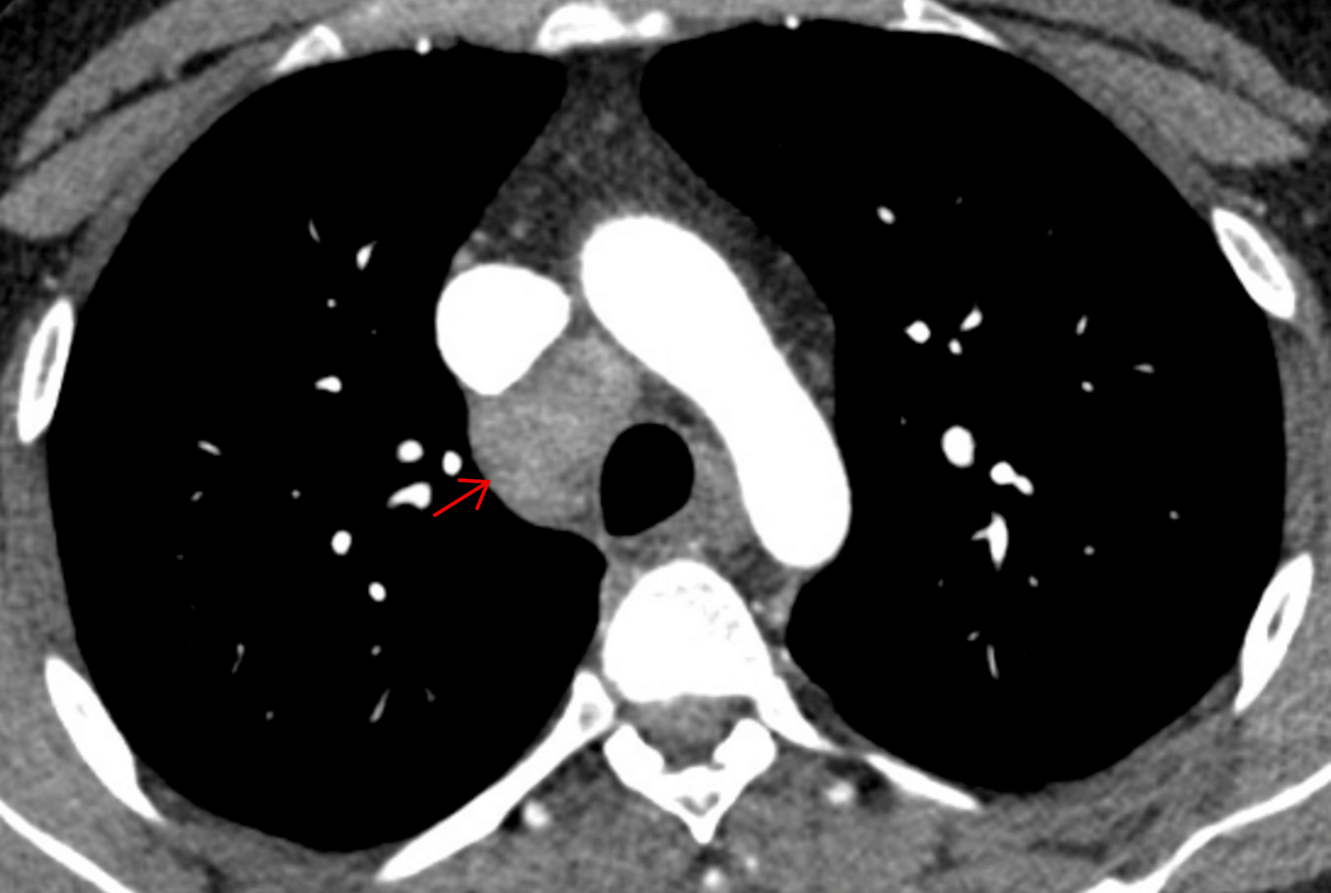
CT thorax showing interval reduction in the size of right paratracheal lymph node CT: computed tomography
